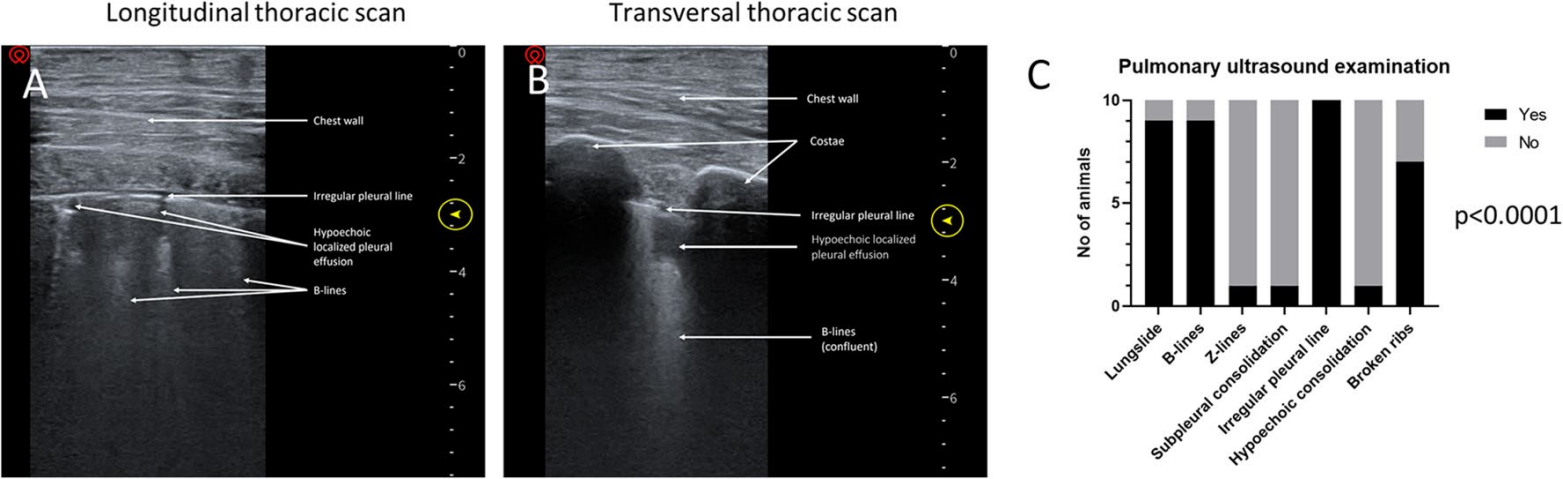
Pulmonary ultrasound examination after lung trauma by the air cannon, confirming changes in lung pathology. None of the changes were detected in the pre-scan. *p* < 0.0001
